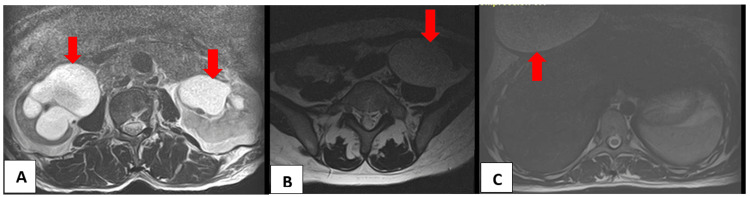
(A) Unenhanced axial T2W MR image of the lumbosacral spine of a 38-year-old man showing bilateral hydronephrosis worse on the right with significant renal cortical thinning. (B) Unenhanced axial T2W MR image of the lumbosacral spine of a 46-year-old woman showing a simple left ovarian cyst. (C) Unenhanced axial T2W MR image at the upper lumbar spine of a 41-year-old woman showing seroma, right breast. All depicted with the red arrows.
